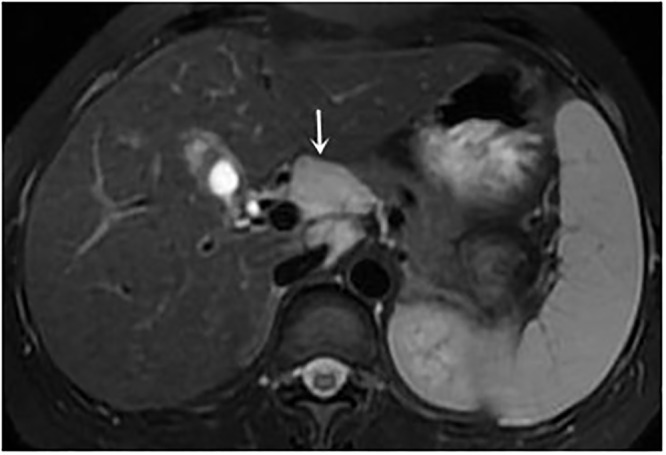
female, 42 years, axial T2-weighted MRI shows lymphadenopathy (*arrow*).
